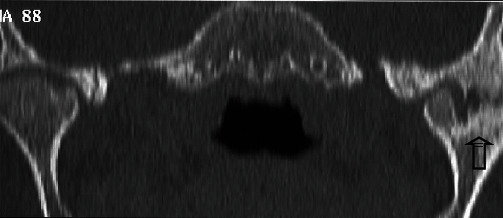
Frontal computed tomography reconstruction in bone rendering showing alteration in articular surfaces of the lateral aspect of the left temporomandibular joint. Note the persistence of joint space in the medial aspect (hatched black arrow).
